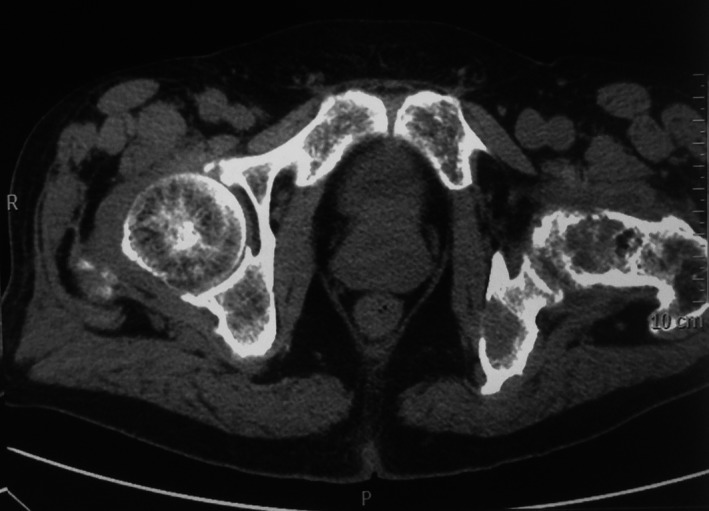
CT scan of the pelvis: multiple lytic bone lesions with cortical rupture and soft tissue invasion.
